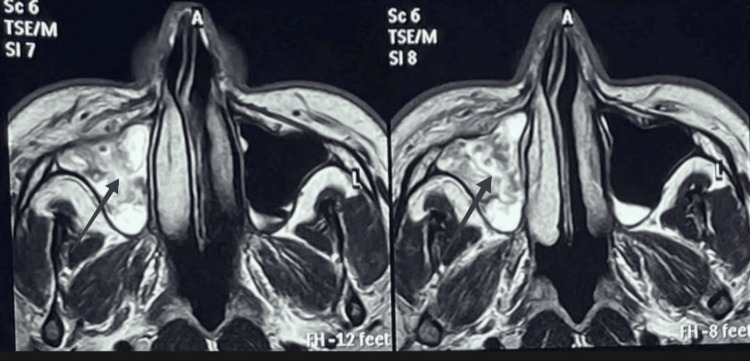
Axial T2-weighted MRI image without contrast showing hyperintense mucosal thickening and intrasinus hypointense contents in the right maxillary sinus.
